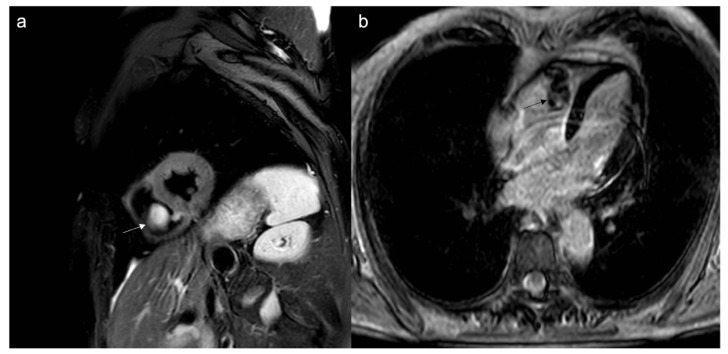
Rhabdomyosarcoma (arrow) is hyperintense on T2-weighted images (a) and it shows contrast enhancement with regions of hypointensity due to central necrosis (b).
